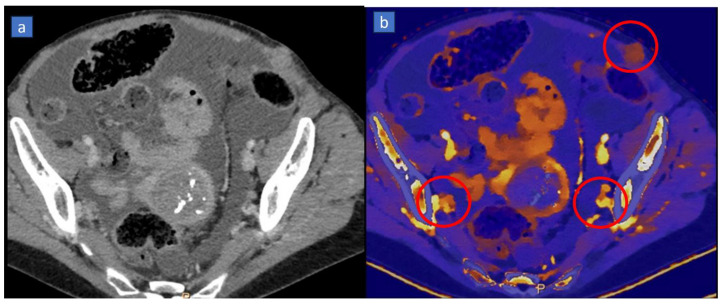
Staging CT in a 49-year-old patient with metastatic ovarian carcinoma. On an axial contrast-enhanced CT image, (a) the presence of ascites allows us to recognized multiple subtle peritoneal nodules. On the color-coded iodine maps, (b) metastatic peritoneal implants (red circles) can be clearly detected because of the avid iodine uptake.
